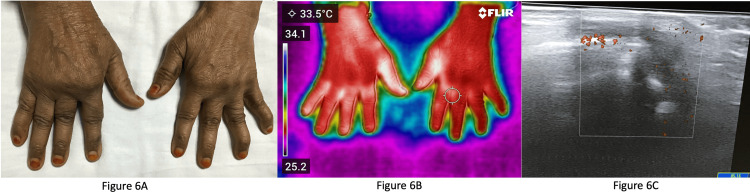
Image of a patient with rheumatoid arthritis (RA) and deformed hands 6A: Clinical image of a patient with deformed RA with volar subluxation at the right metacarpophalangeal (MCP) joint; 6B: Infrared thermography of the same patient showing increased temperature at the right wrist, bilateral first MCP and proximal interphalangeal (PIP), right second, third, and fourth PIP joints; 6C: Ultrasonography showing synovitis with power doppler uptake in the right wrist.
